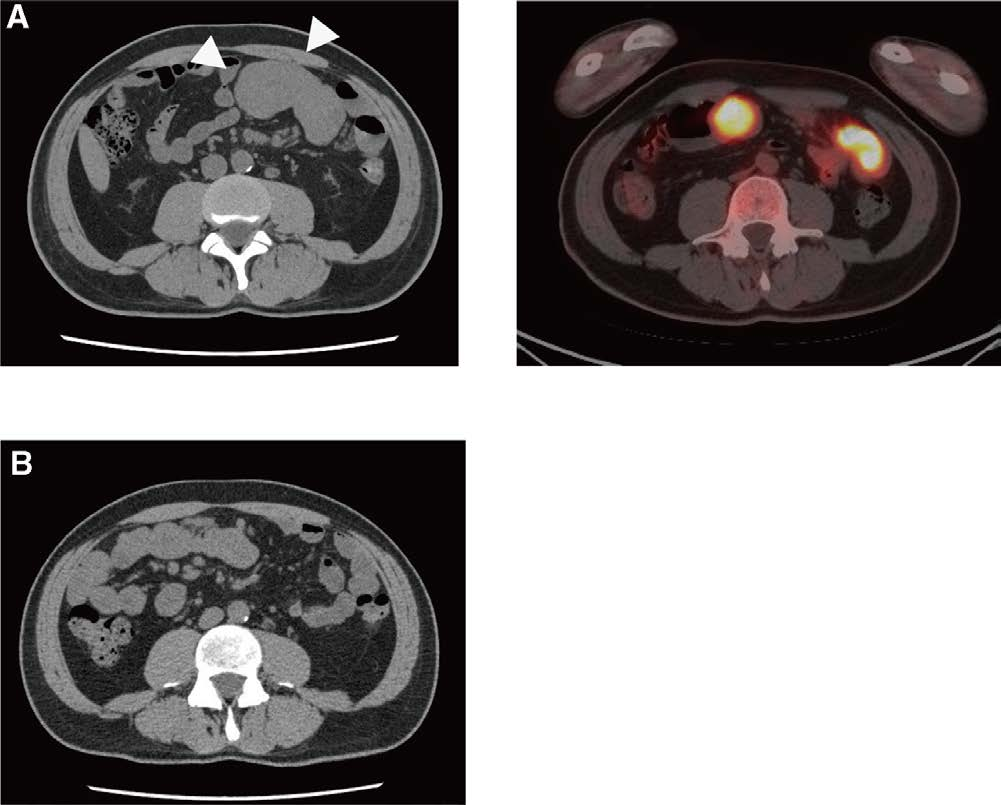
Small intestinal metastasis of pulmonary pleomorphic carcinoma detected by CT (arrowheads; left) and PET-CT (right) (A). CT taken 15 days after initial pembrolizumab administration demonstrated shrinkage of small intestinal metastasis (B). CT = computed tomography, PET-CT = positron emission tomography-computed tomography.
